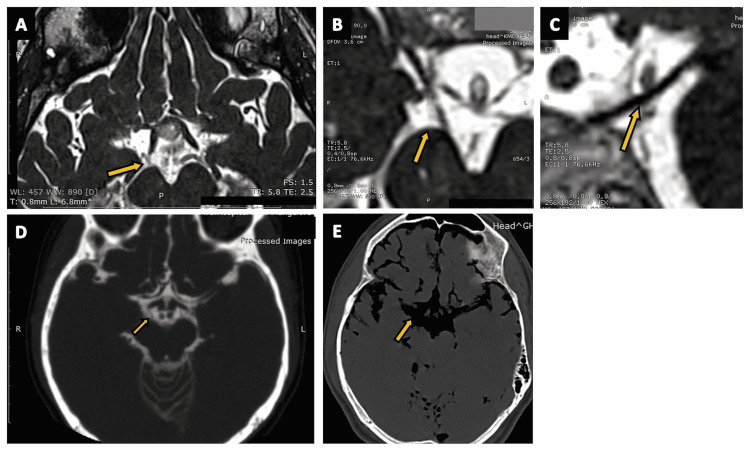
The oculomotor cistern. (A) The oculomotor cistern (arrow) as seen on an axial section of the routine three-dimensional (3D) constructive interference in steady state (CISS) MRI sequence. (B) A zoomed-in version of the oculomotor cistern (arrow) in an axial section of the routine 3D-CISS MRI sequence. (C) The oculomotor nerve (CN III) within the oculomotor cistern (arrow) is seen on an oblique section of the routine 3D-CISS MRI sequence. (D) The oculomotor cistern (arrow) visualized on an axial section of CT cisternography in a case of suspected CSF rhinorrhea. (E) The oculomotor cistern (arrow) as seen on an axial section of CT brain (negative contrast) in a case of pneumocephalus.
